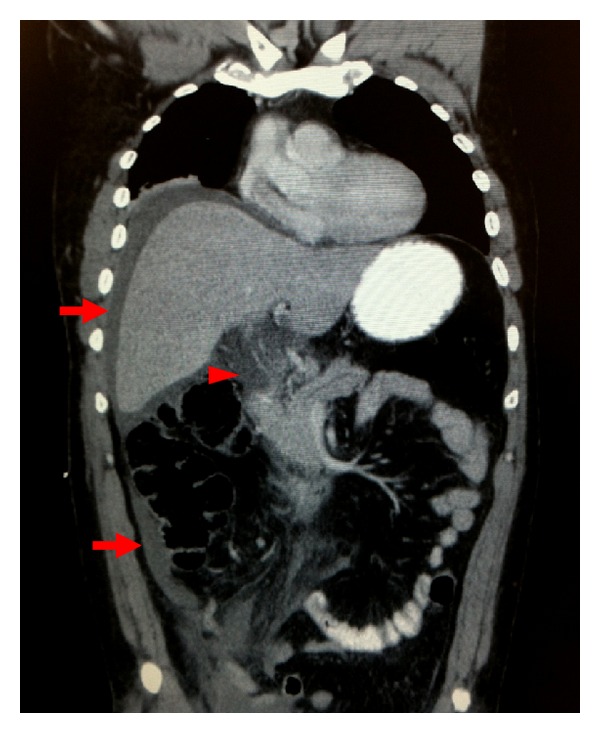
Coronal CT image demonstrating intraabdominal fluid above the liver and along the right pericolic gutter (arrows). “Periportal tracking” is also present (arrowhead), suggestive of an extrahepatic biliary injury. Possible disruption of the extrahepatic bile duct is visualized.
